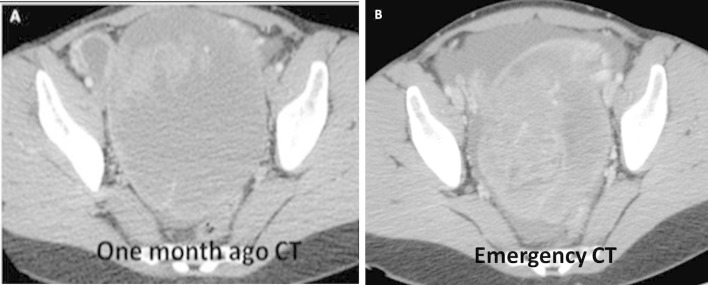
Axial scans which compare staging (A) and emergency CT (B). In emergency CT a
